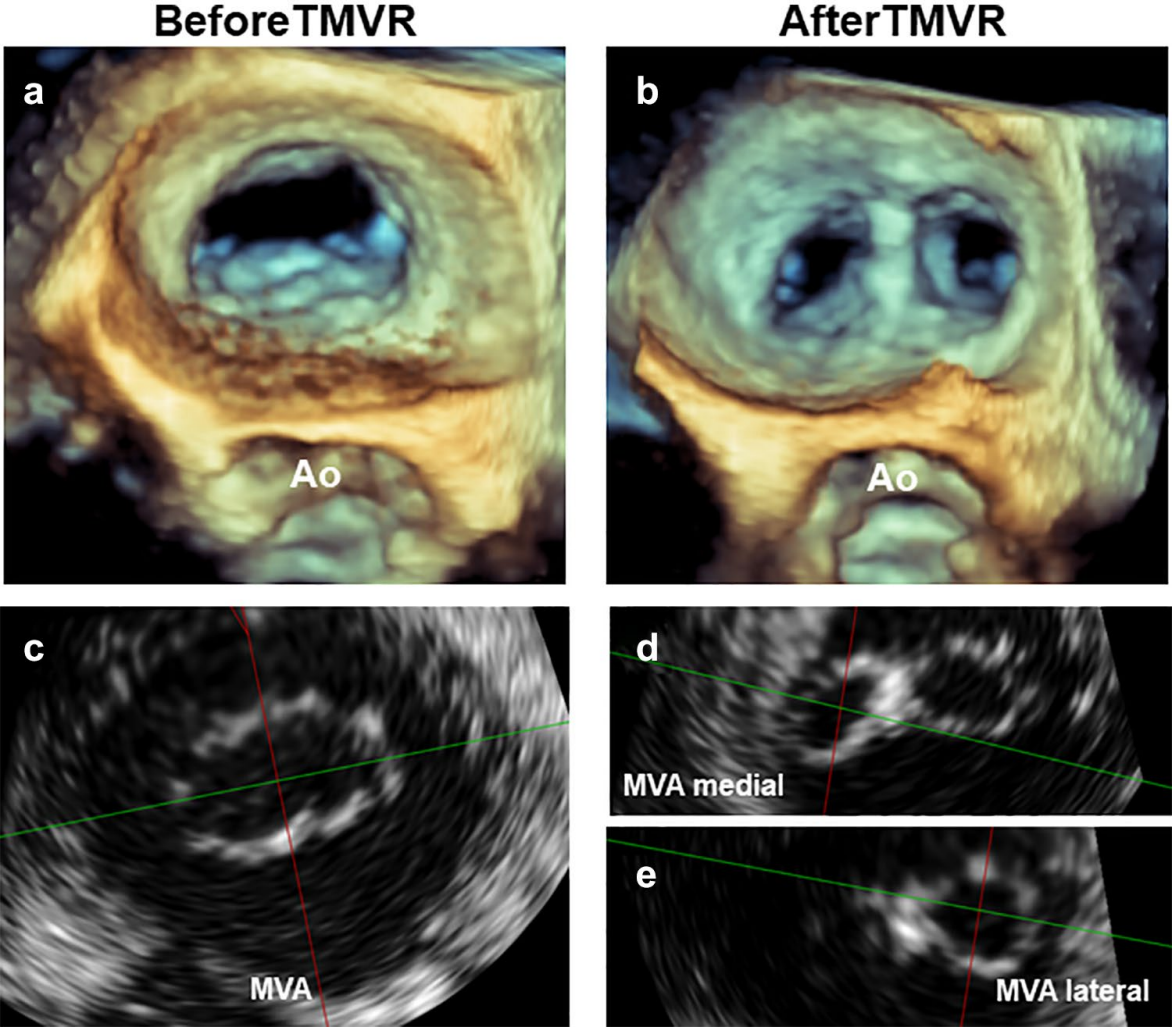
Measurements of mitral valve opening area (MVA) before and after transcatheter mitral valve repair (TMVR). 3D TEE view from the roof of the left atrium in diastole before (a) and after (b) TMVR with direct visualization of the maximum diastolic mitral valve opening area (MVA) before TMVR (c) and the medial and lateral MVA separately after TMVR (d + e). *3D* 3-dimensional, *MVA* indicates mitral valve opening area, *TEE* transesophageal echocardiography, *TMVR* transcatheter mitral valve repair
